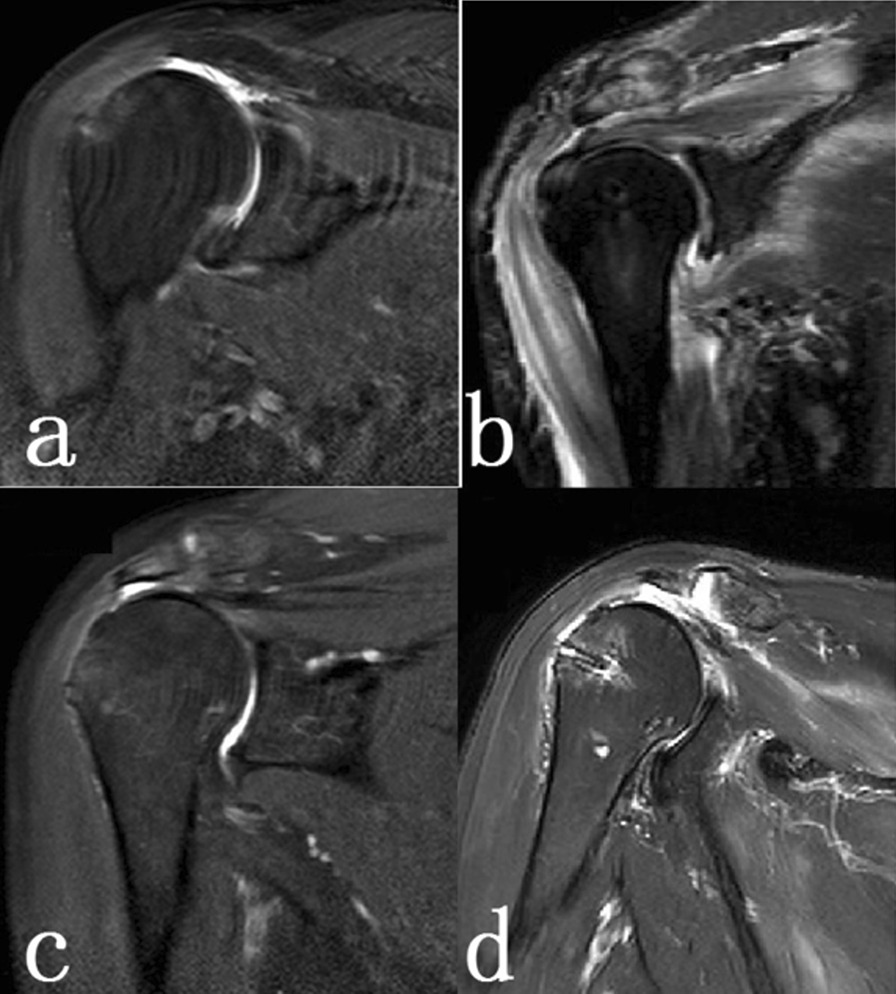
MRI imaging of shoulder reconstruction. a Preoperative complete structural continuity; b postoperative complete structural continuity; c preoperative structural failure; d postoperative structural failure
